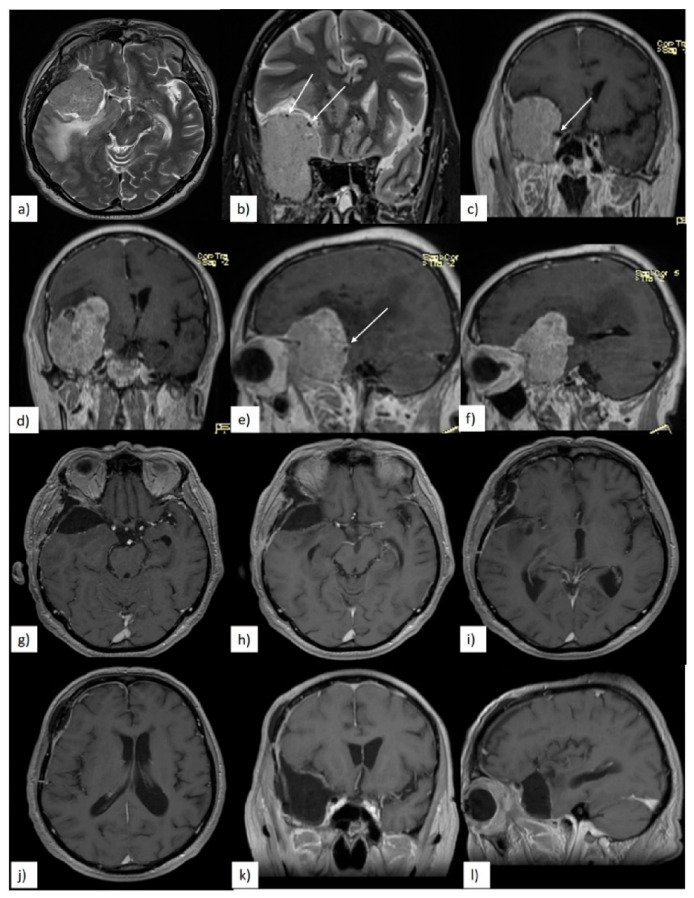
(a–f) Preoperative magnetic resonance imaging (MRI) showing partial arterial encasement of ICA- and middle cerebral artery (MCA)-white arrows; (g–l) 3-month postoperative T1 contrast MRI showing gross total resection (GTR) of the tumor.
